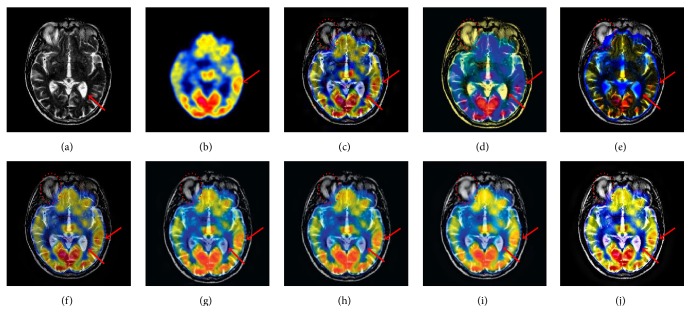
The fusion results of different fusion methods for the MRI and PET images: (a), (b) Source images; fused images by (c) DWT, (d) PCA, (e) IHS, (f) GP, (g) CT, (h) NSST, (i) NSST-1, and (j) the proposed method.
